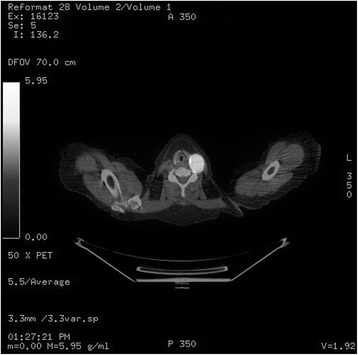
Combined CT/PET capture of fluorodeoxyglucose (FDG) uptake in left lateral neck mass
